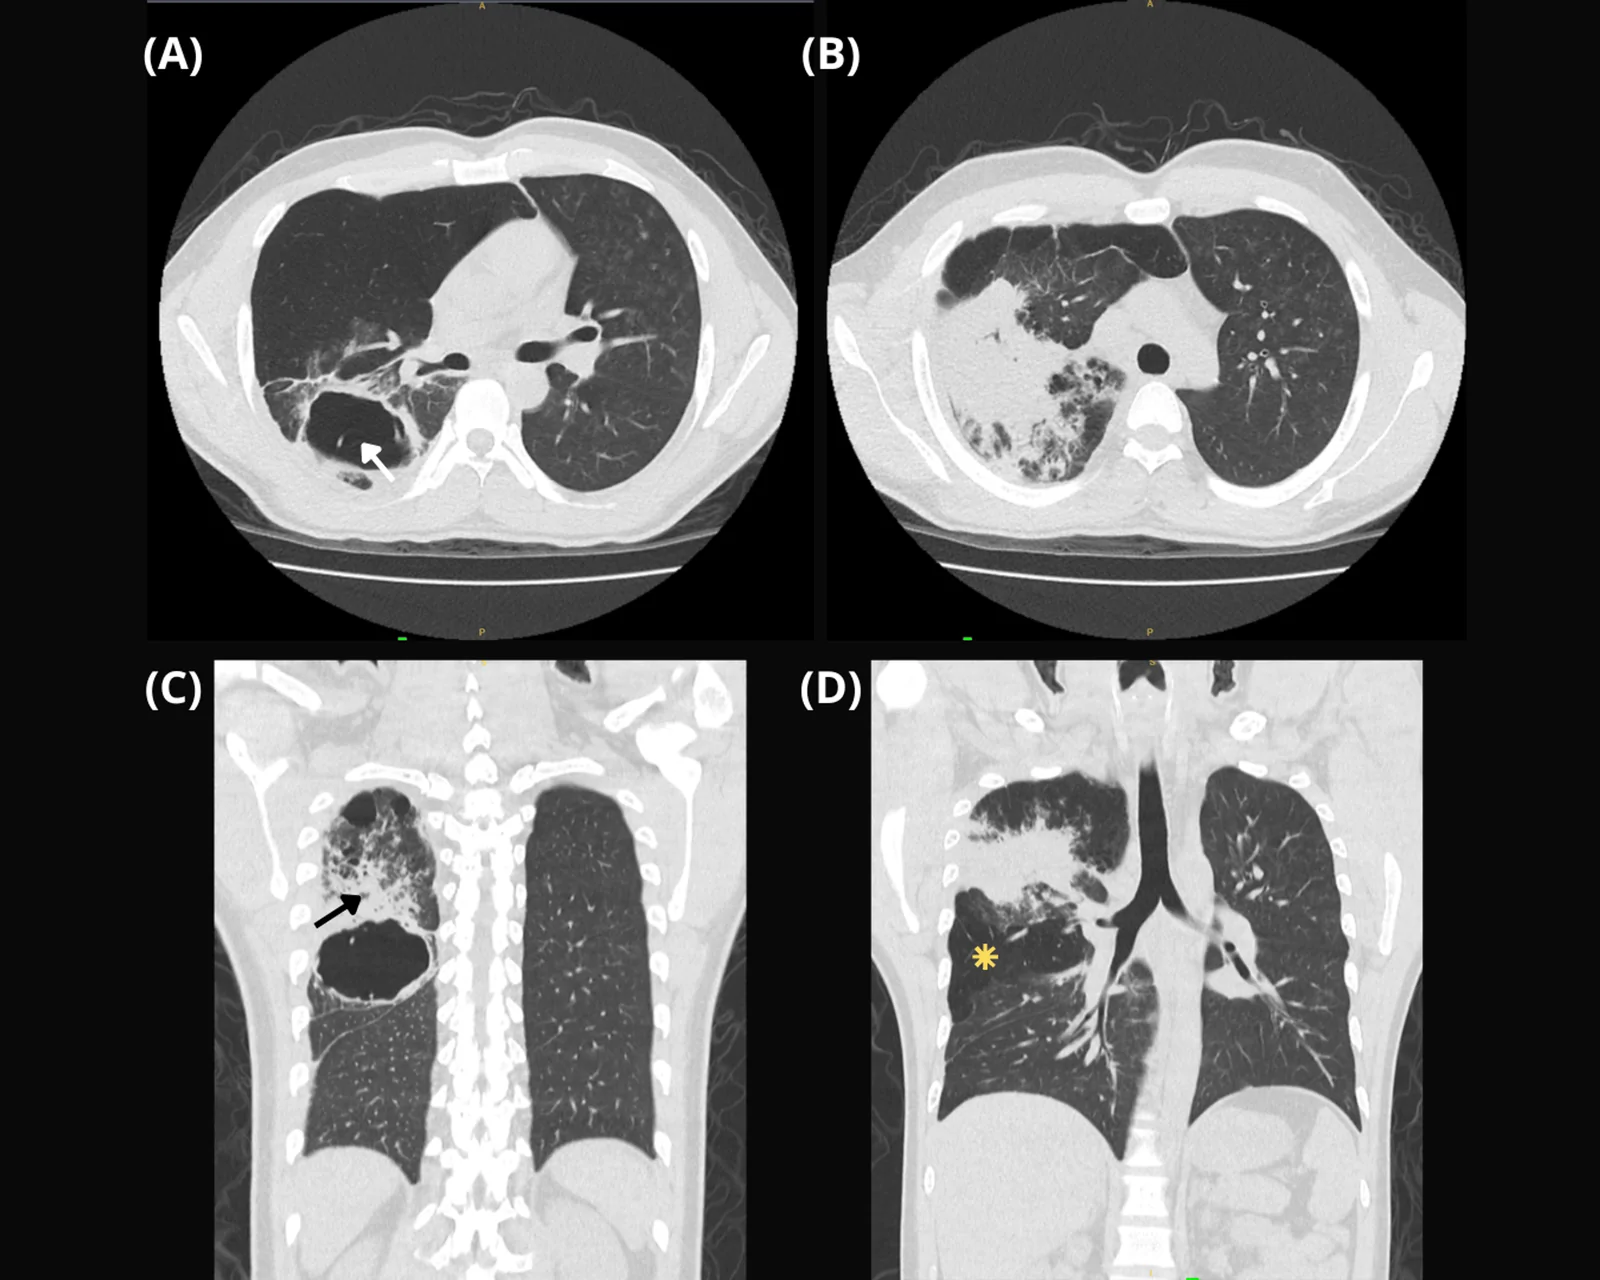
Contrast-enhanced chest computed tomography on admission. Axial (A, B) and coronal (C, D) images demonstrate right upper lobe consolidation (black arrow) associated with a thin-walled cavitary lesion containing an air-fluid level (white arrow), consistent with a lung abscess, together with hyperinflation of the adjacent right middle lobe (*).
